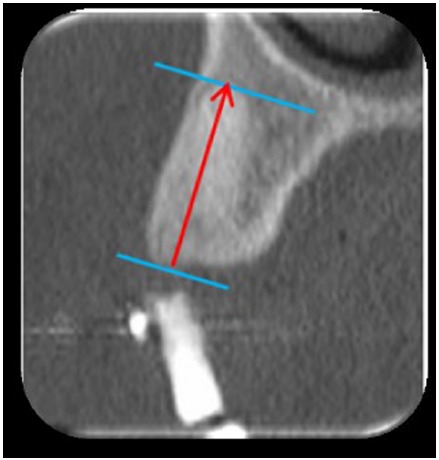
Three months after extraction, height is evaluated as the distance between apex and lowest crestal visible point on the postoperative CT Scan.
